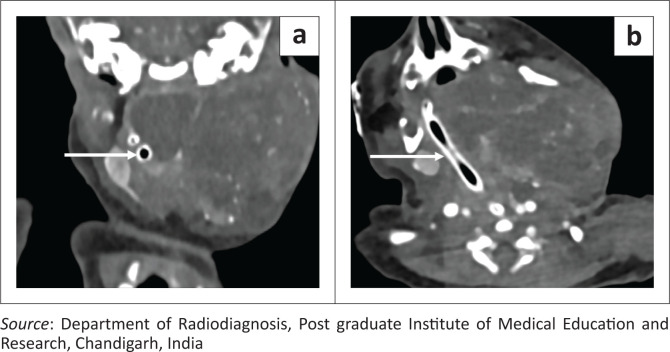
Coronal (a) and axial contrast-enhanced CT images (b) demonstrate a hypodense cystic lesion in the left neck, extending medially into the nasopharynx and causing marked airway compromise. Laterally, the lesion produces focal contour bulging of the overlying skin and involves the parotid and submandibular spaces. The tracheostomy tube is compressed and displaced by the mass (*arrows*).
